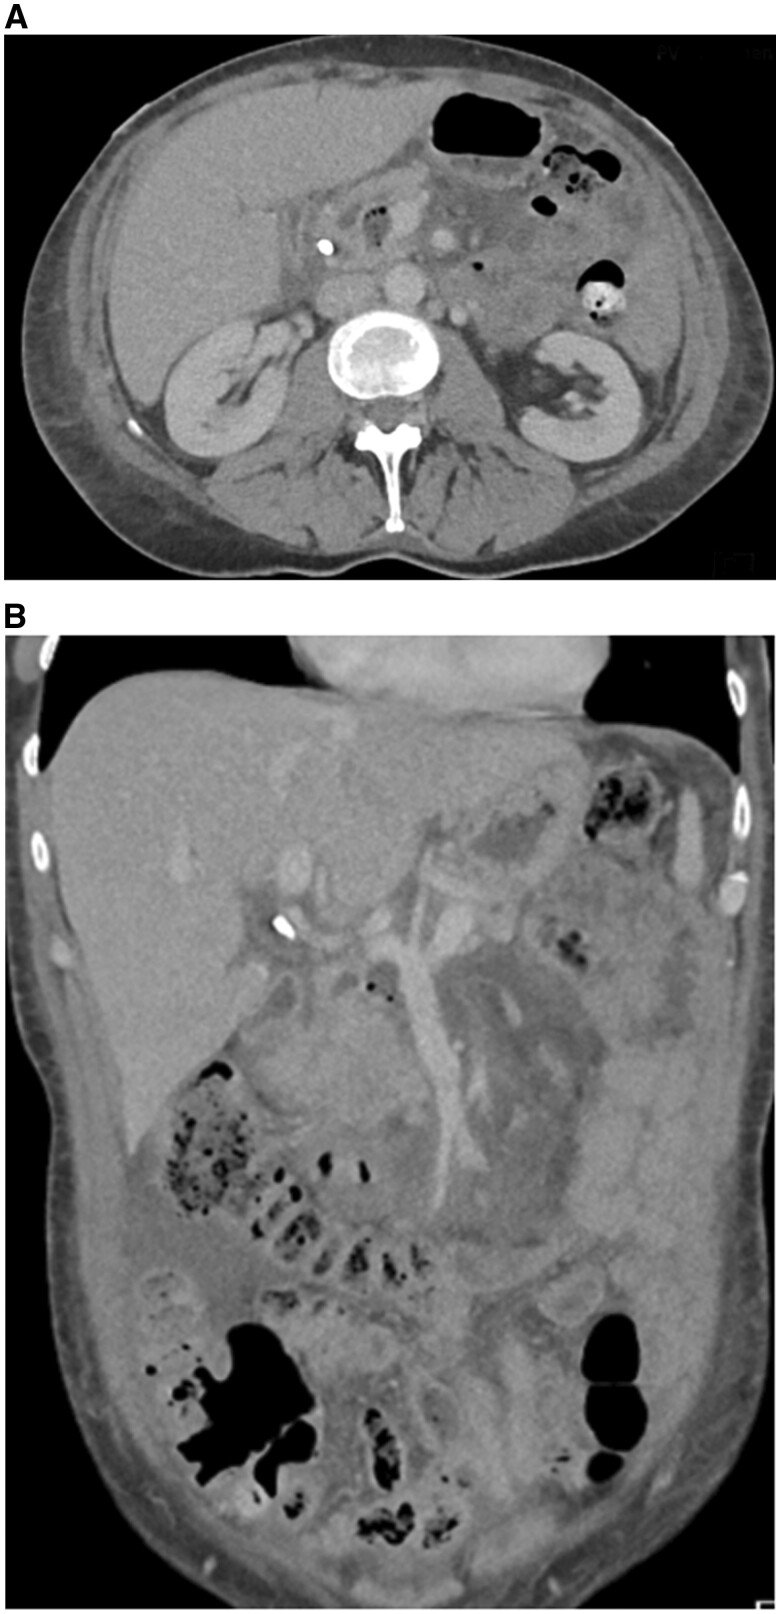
(A) Pancreatic head collection and surrounding inflammatory changes. Portal venous contrast-enhanced CT axial image of the upper abdomen demonstrates a collection at the pancreatic head containing gas locules consistent with pancreatic duct perforation. No pancreatic necrosis. Extensive inflammatory changes in peripancreatic tissues and mesentery. Common bile duct stent *in situ*. (B) Pancreatitis with surrounding inflammatory changes and free fluid. Portal venous contrast-enhanced CT coronal image demonstrates inflammatory change and free fluid centred on pancreas consistent with pancreatitis. Common bile duct stent *in situ*.
